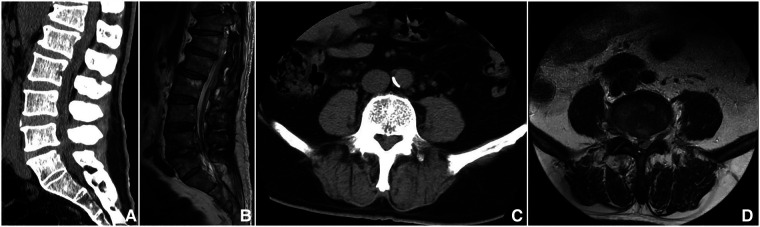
A 57-year-old male, whose complaint was intractable low back pain for 1 year and lower limb pain for 1 month. (A,B) Sagittal CT and MRI showed an epidural abscess compressing the thecal sac at L4–5. (C,D) Axial CT and MRI showed the epidural abscess.
